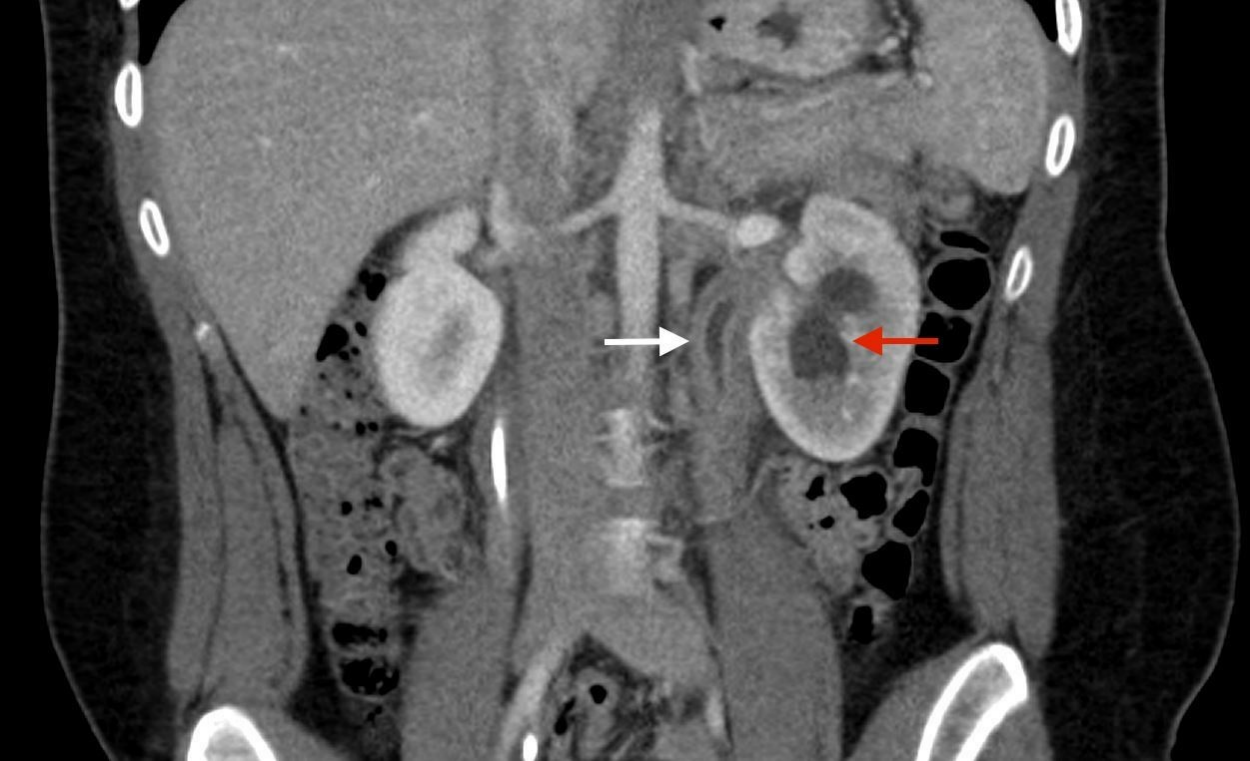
Coronal CT image showing thickened left ureter (white arrow) and moderate hydronephrosis of the left kidney (red arrow) CT: computed tomography
